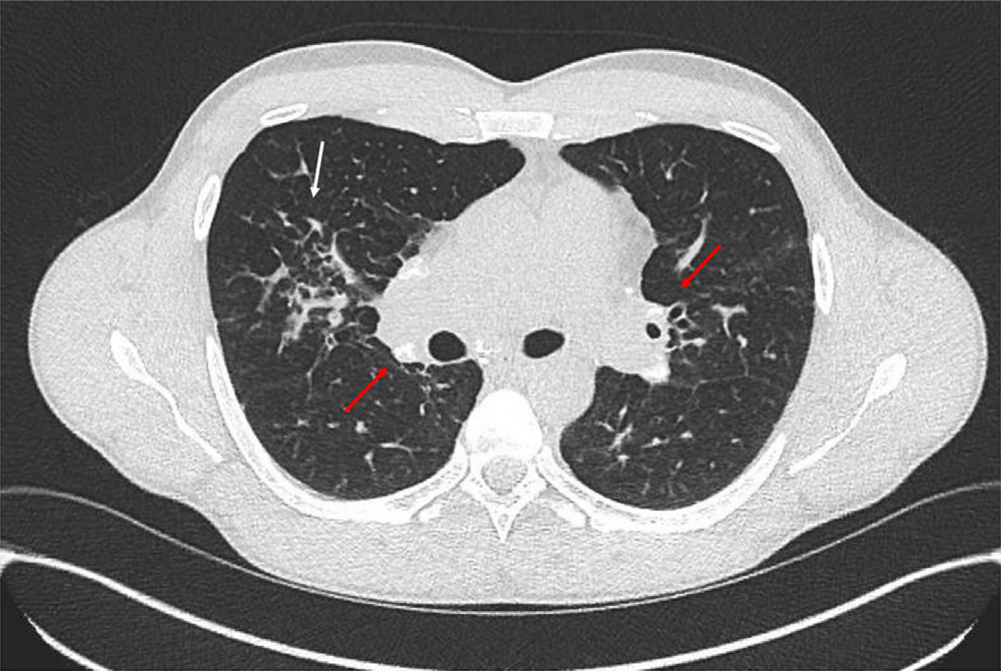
Typical appearances on HRCT. Typical appearances of thoracic sarcoidosis on HRCT: calcified hilar and mediastinal nodes (red arrows), and parenchymal nodularity (white arrow).
